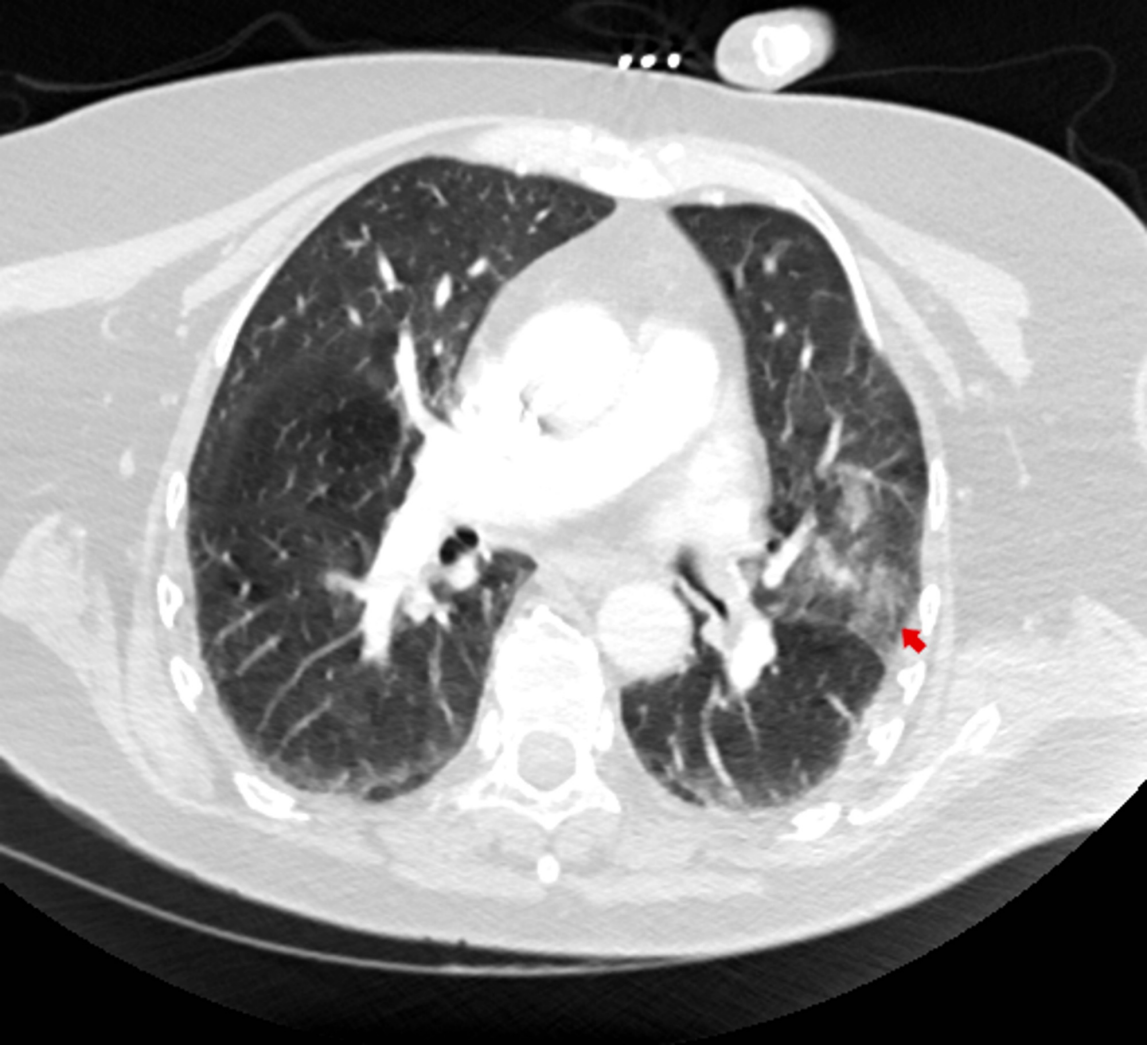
Computed Tomography Angiography for Pulmonary Embolism (CTA PE) There is no evidence of pulmonary embolism but there are ground-glass opacities compatible with left lower lobe pneumonia (red arrow)
